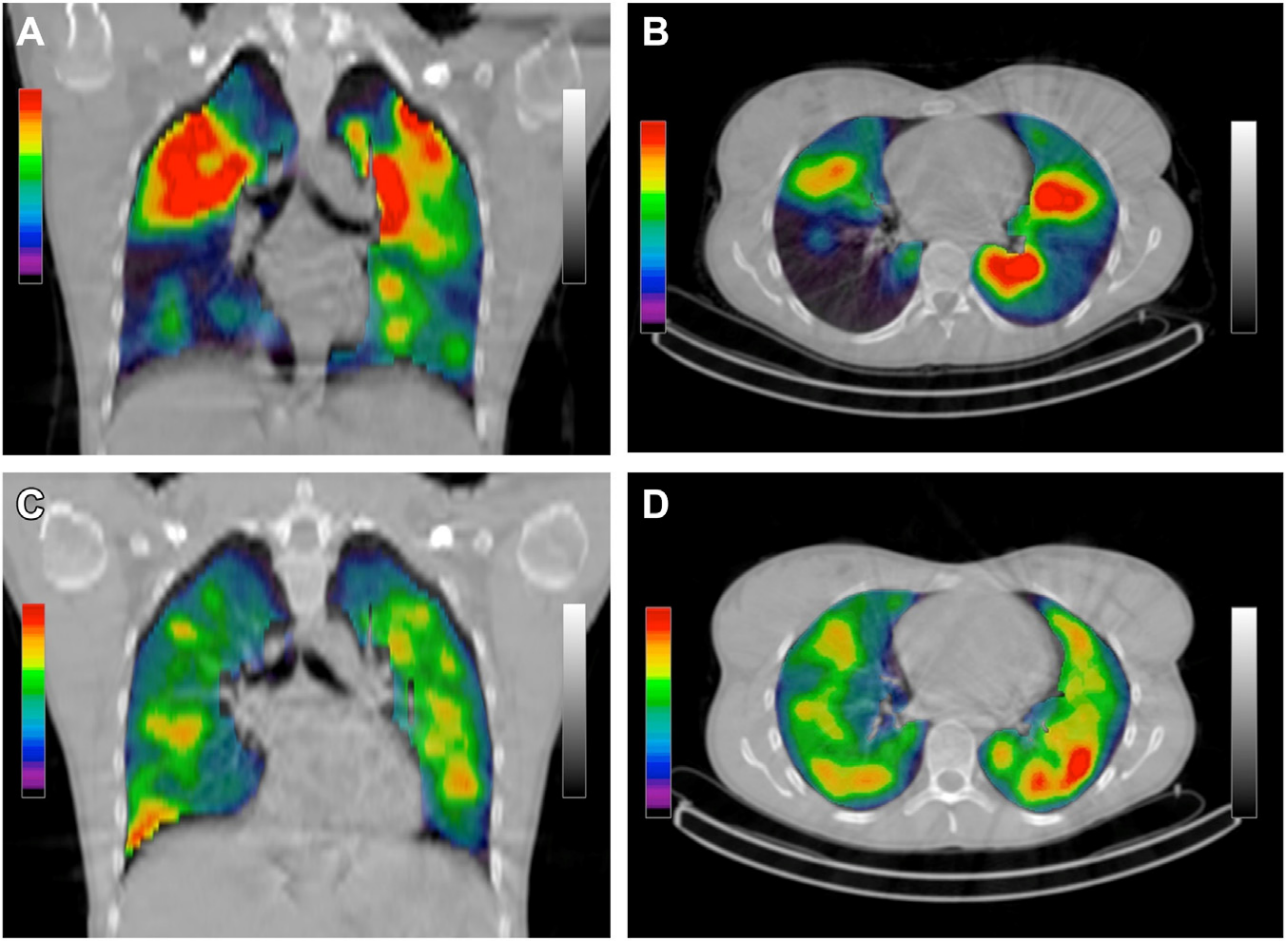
Technegas ventilation SPECT image showing the distribution of ventilation in upright posture, before and after bronchodilator inhalation. Female subject aged 28 years with asthma. A and B, Baseline images during bronchoconstriction (FEV_1_ of −1.99 *z* scores). C and D, Postbronchodilator images after improvement in FEV_1_ of 28% of baseline value.
